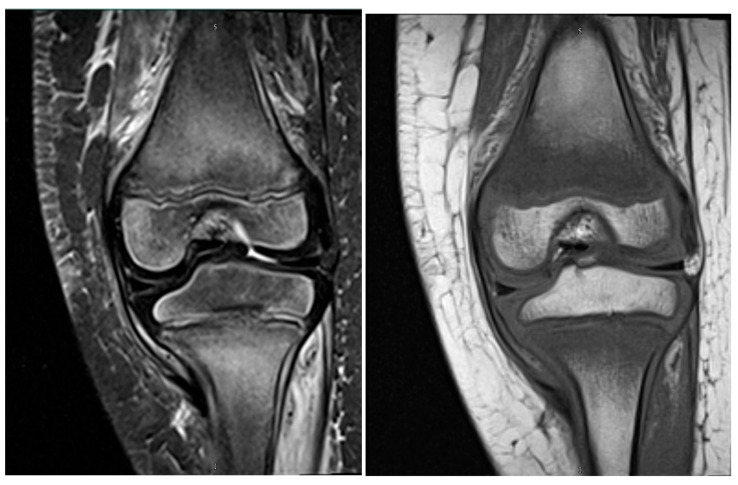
MRI of lower extremities shows abnormal STIR signals with metaphyseal/physeal involvement, periosteal reactions, and small joint effusions (left). Hypointense T1-weighted images depicting the same are present (right).
